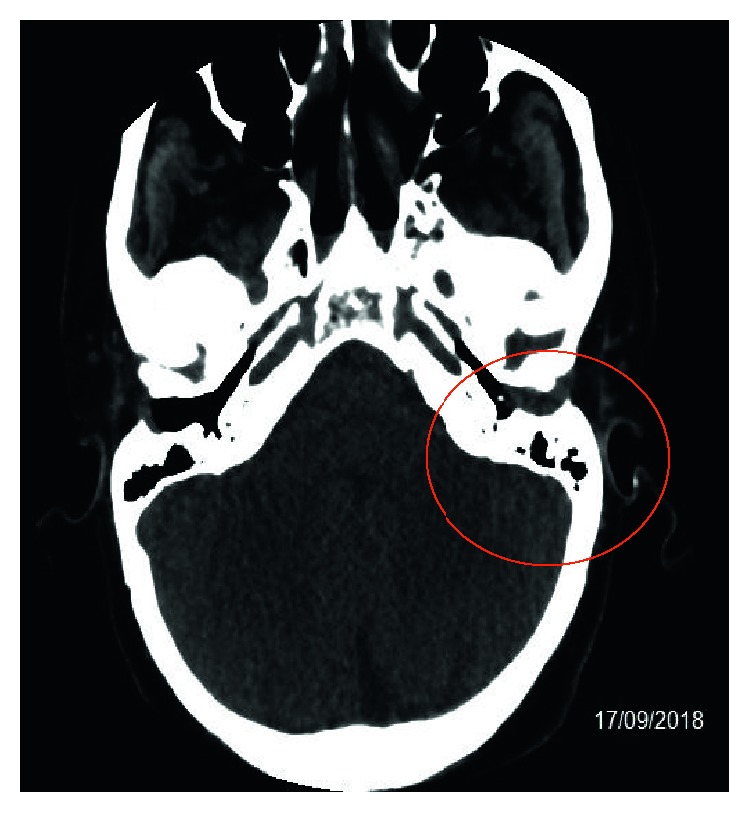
CT of the left ear without contrast medium.
